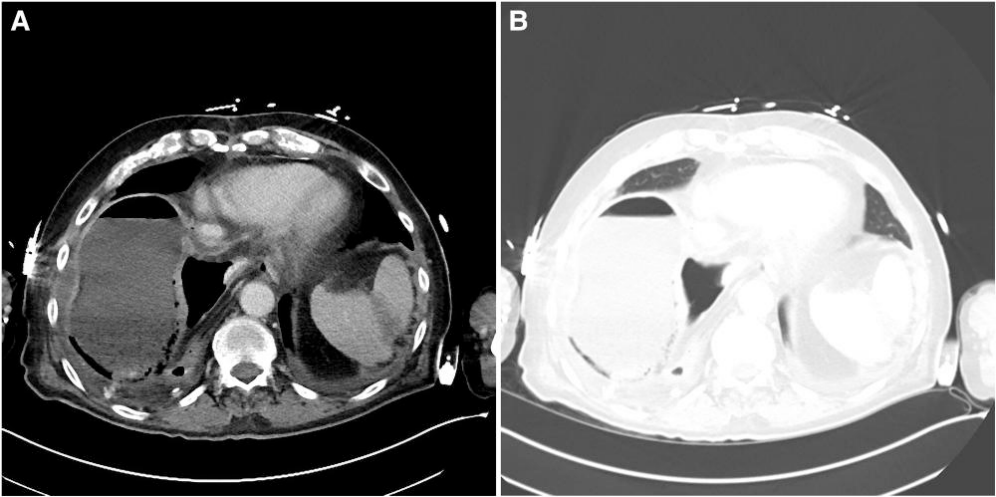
A*, Axial contrast-enhanced CT at the level of lung bases. The patient has a remote history of esophagectomy with gastric pull-through, lending to his altered anatomy. Foci of gas are seen within the gastric wall, consistent with gastric pneumatosis. *B*, Axial contrast-enhanced CT viewed in the lung window to better visualize tissue density and distinguish air-filled space from fluid-filled space. Abbreviation: CT, computed tomography.
